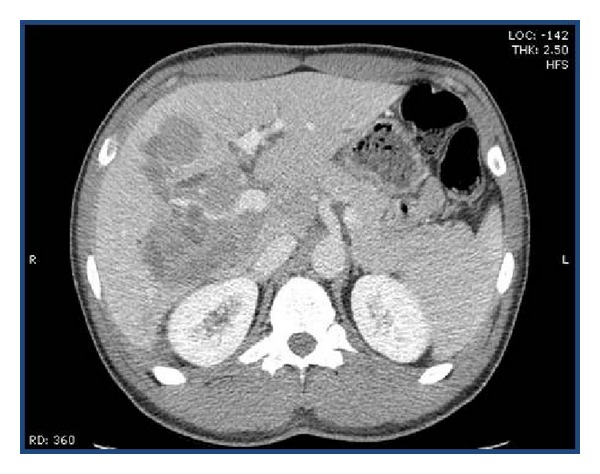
CT scan with intravenous contrast-portal venous phase showing multiple lesions in the liver.
